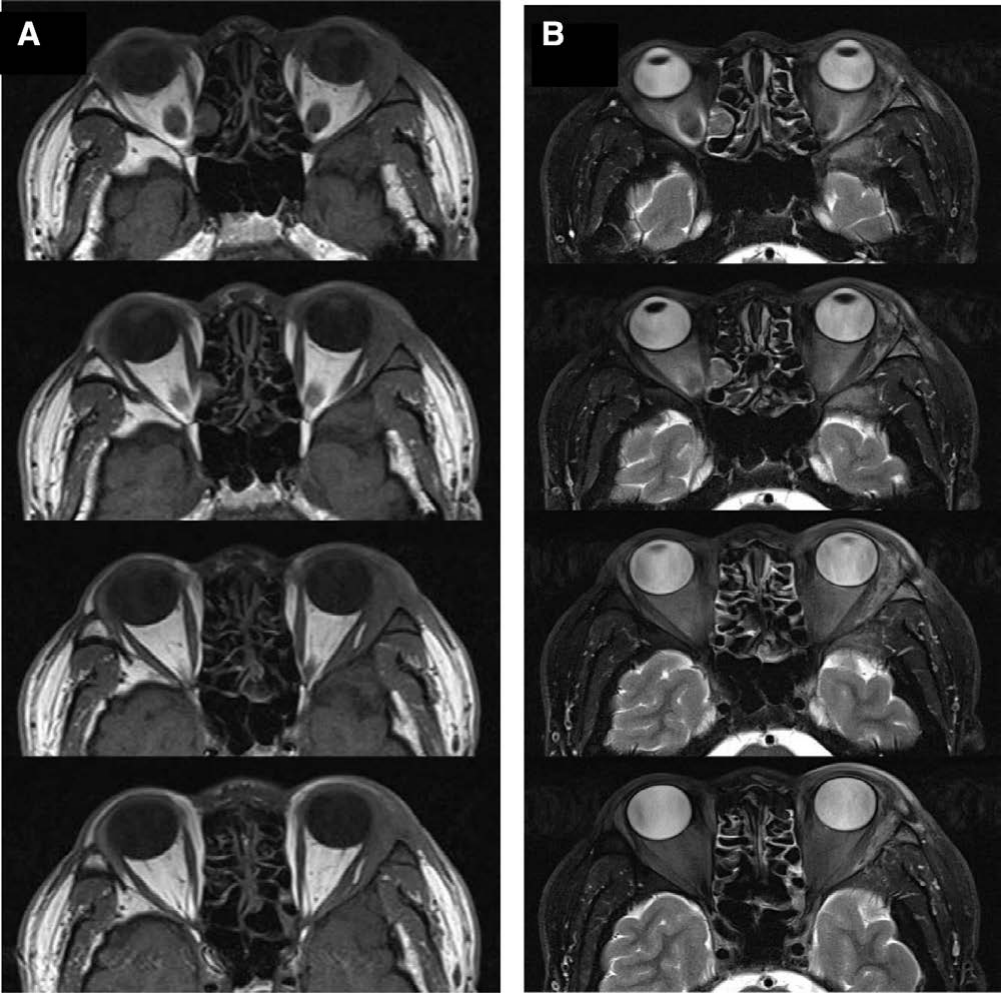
T1-weighted axial (A) and T2-fat suppressed axial (B) magnetic resonance image showing left orbital mass in the area of the lacrimal gland (bony wall of orbit was infiltrated, the periosteum was intact).
